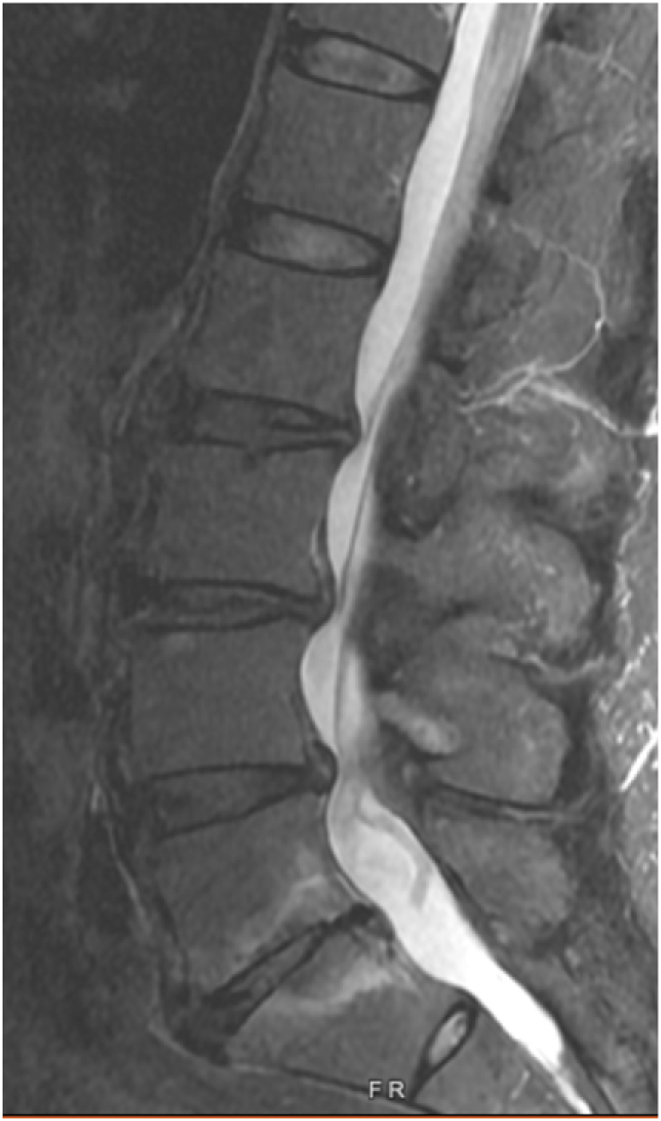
Sagittal STIR MRI pre-procedure.
